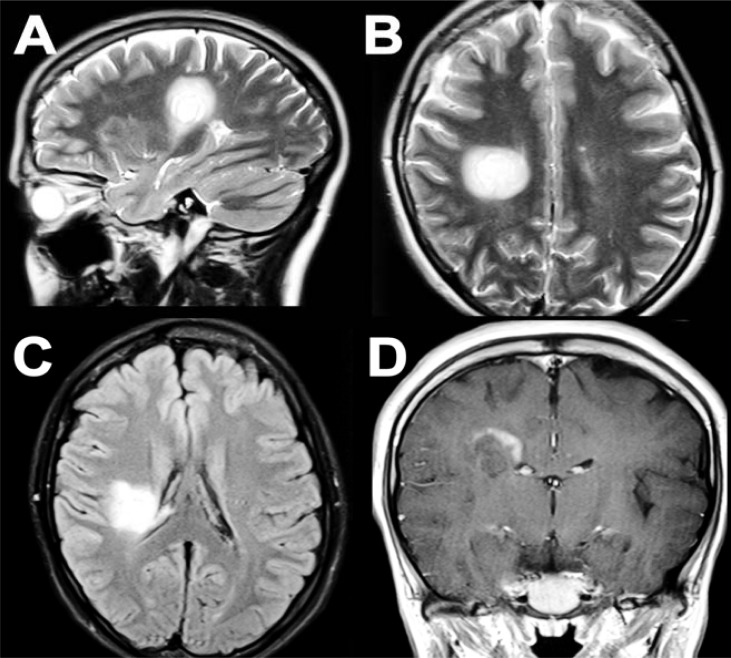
Sagittal and axial T2-weighted brain MRI showing hyperintense concentric onion-like mass in the right parieto-frontal periventricular white matter (A,B), axial FLAIR MRI showing hyperintense concentric pattern in the right parieto-frontal periventricular white matter (C), and coronal post-contrast T1-weighted image demonstrating some open ring enhancement of the lesion in the right parieto-frontal area
